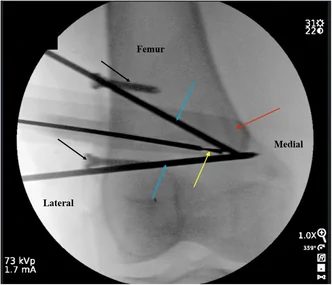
Placement of radiolucent retractor for osteotomy. Right knee, anteroposterior fluoroscopic image taken with patient in supine position. Temporary holding set screws (black arrows) and 2.4 mm Kirschner wires (blue arrows) secure the 3D patient‐specific cutting guide on the lateral aspect of the knee as the oscillating saw (yellow arrow) is introduced through the cutting guide. Position of the wide radiolucent retractor placed posteriorly behind the distal femur (red arrow) should be in line with the direction and position of the oscillating saw (yellow arrow) on AP fluoroscopic radiographs as it is crucial for protecting the posterior neurovascular structures. (3D, 3‐dimensional; AP, anteroposterior.)
